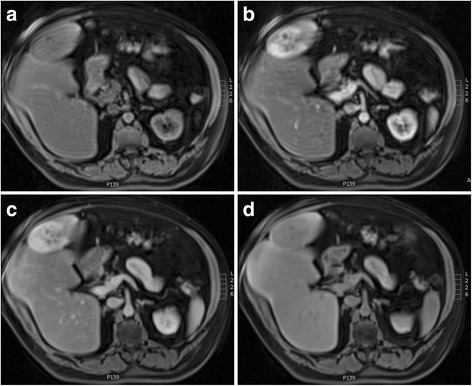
MRI T1WI after contrast. a Arterial phase. b Portal venous phase. c Delayed 2 min. d Delayed 10 min
